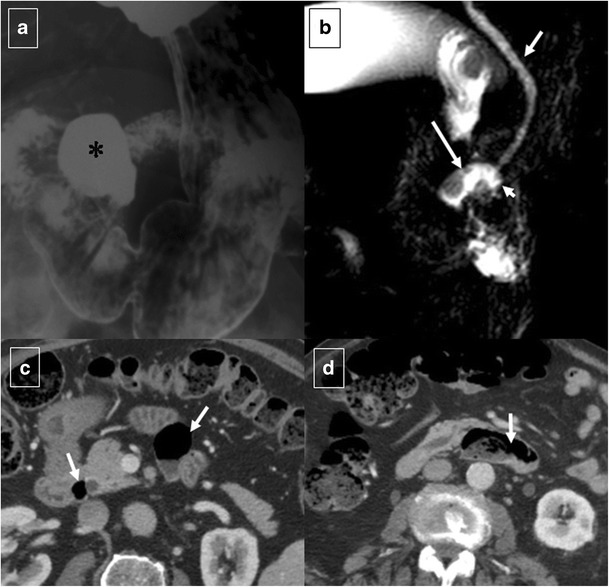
Duodenal diverticula in different patients. a Anteroposterior image from a single-contrast upper gastrointestinal series shows a typical outpouching (*) arising from descending duodenum. b Magnetic resonance cholangiopancreatography shows a heterogeneous outpouching (large arrow) in the papillary region. Note common bile duct (arrow) and proximal Wirsung duct (arrowhead). c Axial CT demonstrates two duodenal diverticula (arrows) in the periampullary region (air-filled) and fourth portion of the duodenum (with air-fluid level). d Axial CT reveals a diverticulum (arrow) that contains debris in the third portion of the duodenum. Air bubbles inside the lesion are virtually pathognomonic for a duodenal diverticulum
